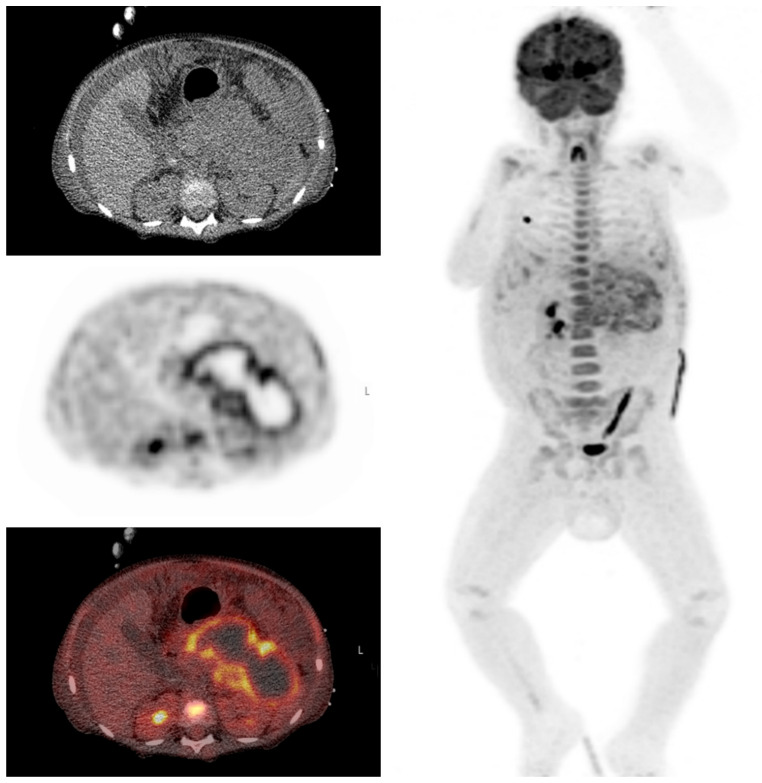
Clinical example 3. This scan shows an irregular hypodense lesion in the pancreatic region on low-dose CT and a photopenic area with hot rim on FDG-PET.
